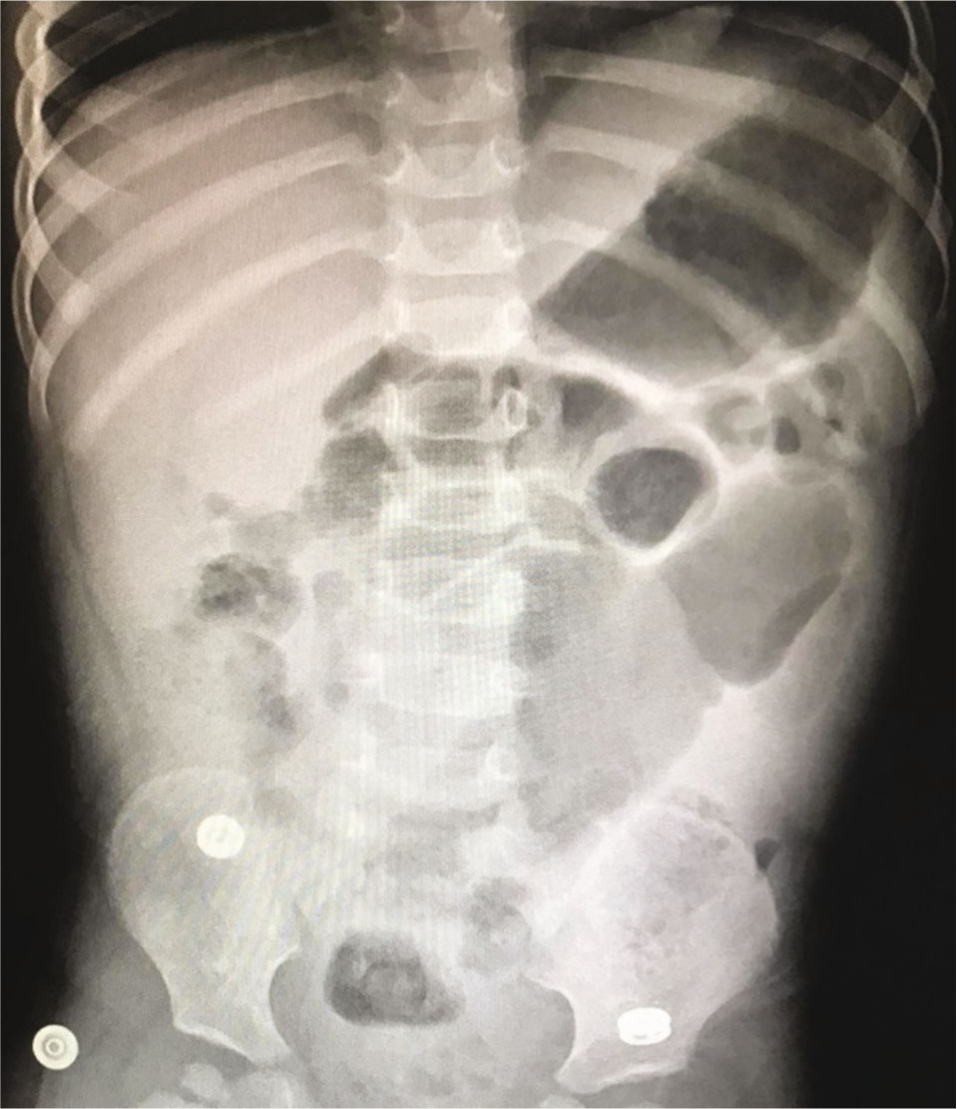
Preoperative abdominal X-ray shows foreign body ingestion though there was no suggestive history.
